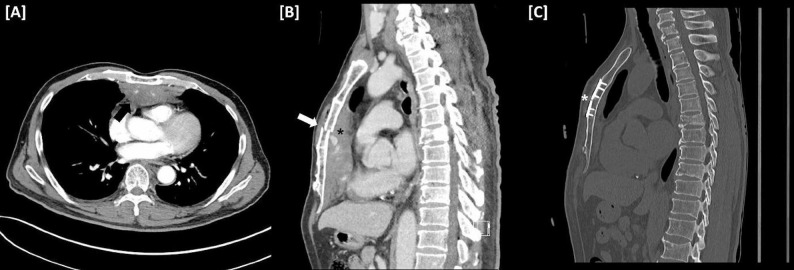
Chest CT scans. [A] Preoperative axial images showing an anterior mediastinal hematoma (black arrow). [B] Sagittal reconstruction demonstrating a displaced sternum (white arrow) with retrosternal hemorrhage (black asterisk). [C] Postoperative follow-up CT showing proper sternal alignment (white asterisk) and resolution of the hematoma
